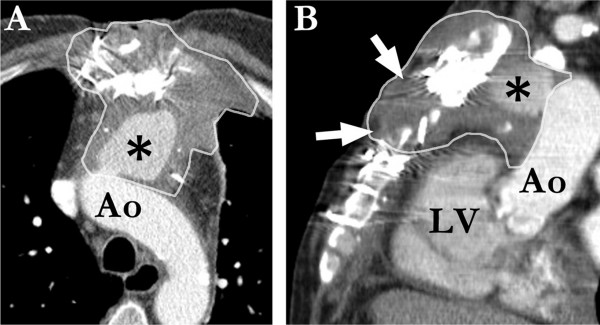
DSWI with pseudoaneurysm. CT axial (A) and sagittal (B) reconstructions show clearly contrast-enhancing soft tissue in the upper and anterior mediastinum (areas encirceled by white lines); ascending aorta (Ao), pseudoaneurysm (asterisk), left ventricle (LV) and massive sternal bone defects (white arrows).
